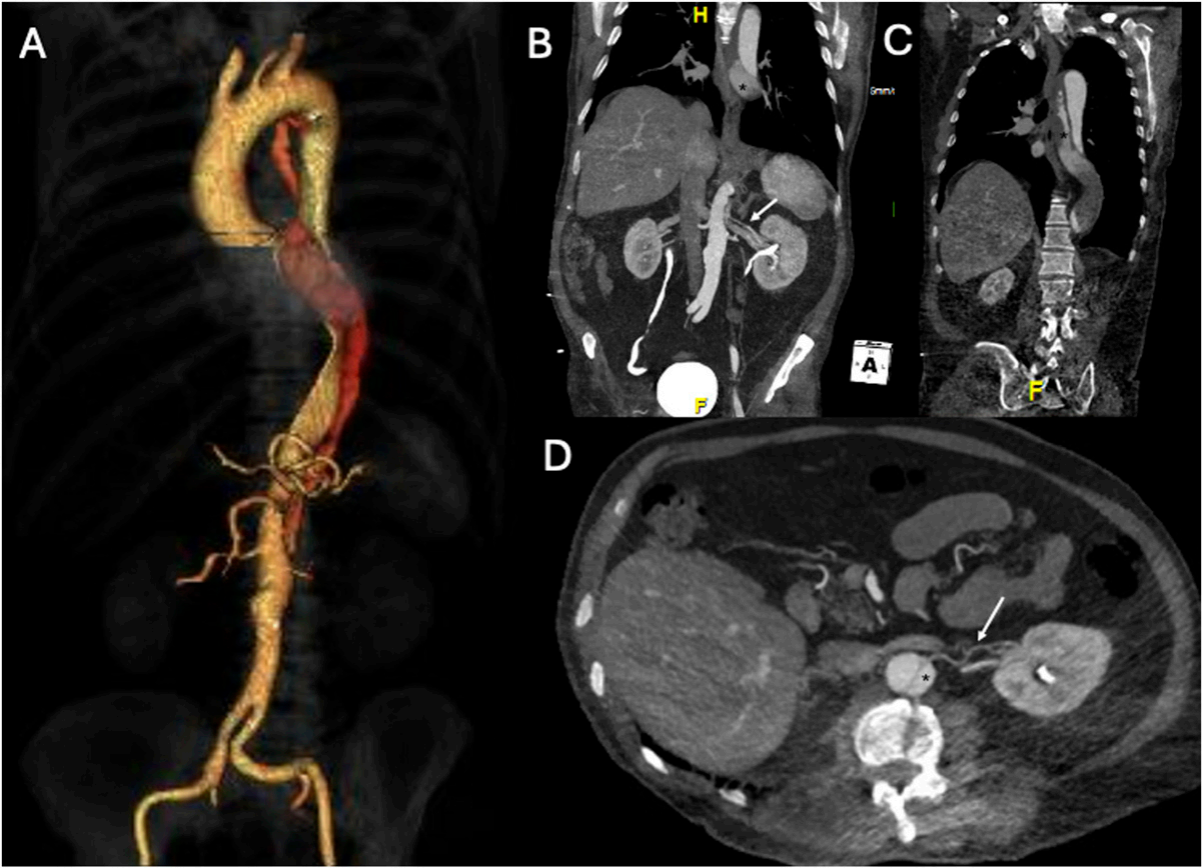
April 2022 pre-operative three dimensions volume rendering (3D VR) and Multi-Planar Reconstruction (MPR) Computed Tomography Angiography (CTA) of Type B aortic dissection (TBAD); (A) 3D VR of total aorta; (B-C-D) MPR CTA showing both false lumen (FL, star) and left accessory renal artery (ARA, arrow) in three different images: (B) MPR in an oblique coronal plane showing the entry tear (ET) in the abdominal aorta (zone 8) in direct communication with left ARA (arrow) and the FL perfusion at this level (star), (C) MPR in an oblique coronal plane showing FL patency up to zone 4 (star), (D) MPR in an oblique axial plane showing the left ARA (arrow) in direct communication with the ET.
